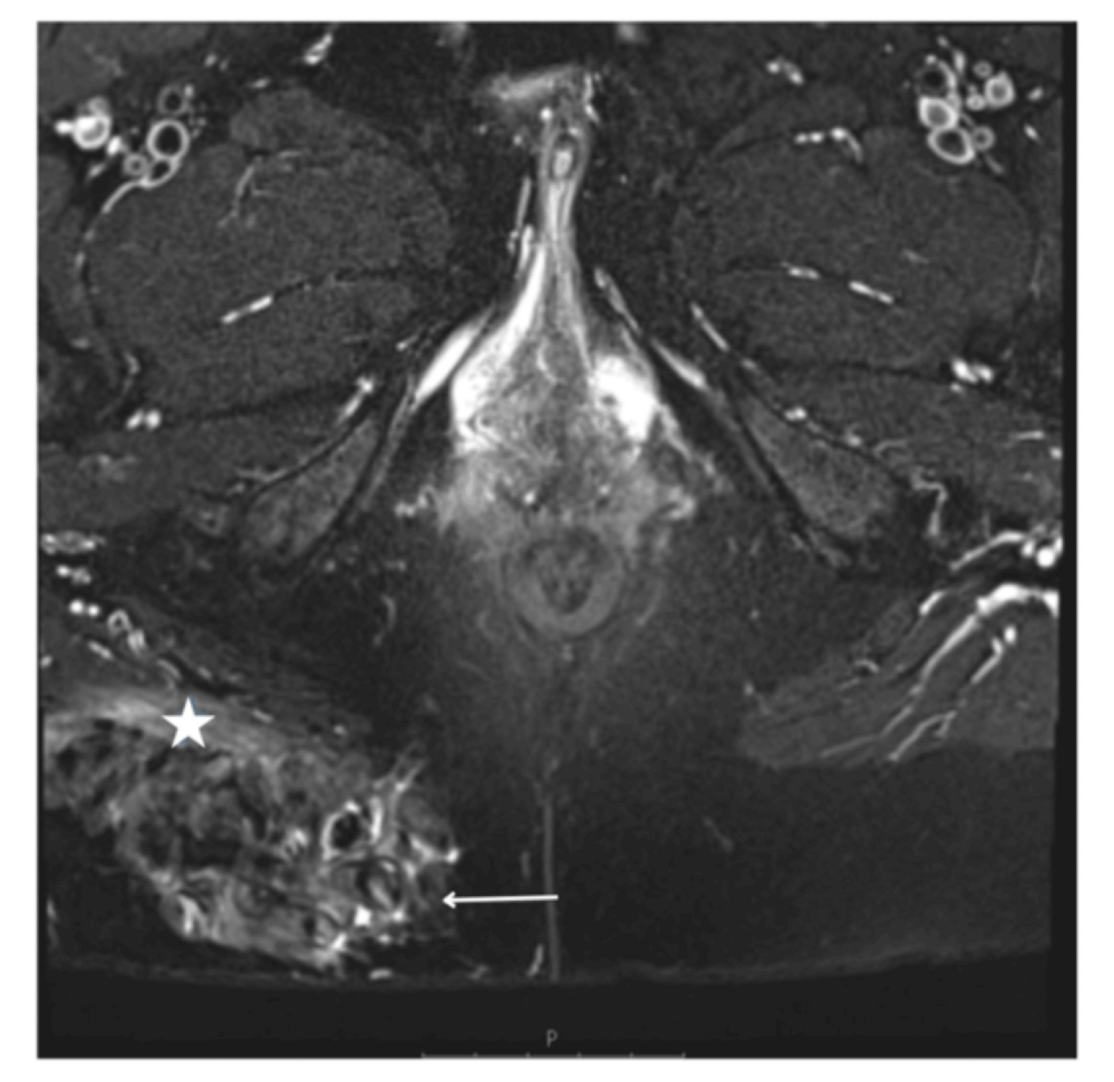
Axial T2 fat sat MRI image of the pelvis. Inflammatory stranding and thickening surrounding fat lobules with the right buttock (arrow). Inflammatory, oedematous signal also involving the adjacent right gluteal muscle fibres (asterisk)
